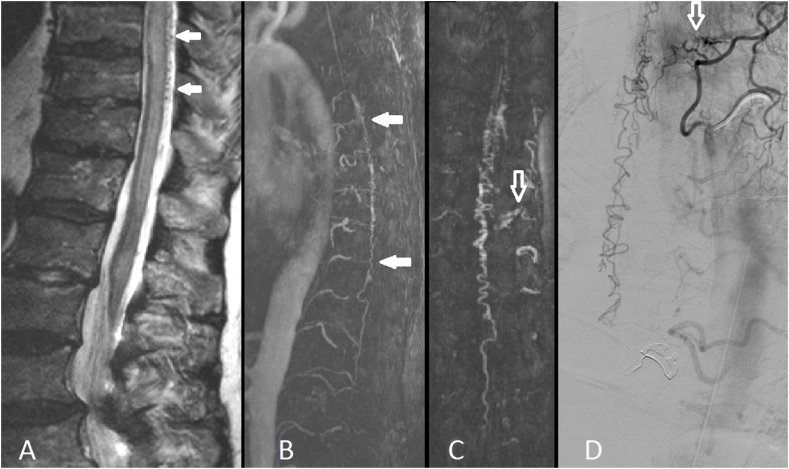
75-year-old male presenting with a SDAVF. Spinal MRI (A) demonstrates spinal cord edema with congested and dilated tortuous intradural veins surrounding the spinal cord. Additional 4D-MRA (B,C) showed a left sided SDAVF at the level of Th5-Th6. The SDAVF was confirmed by DSA (D).
